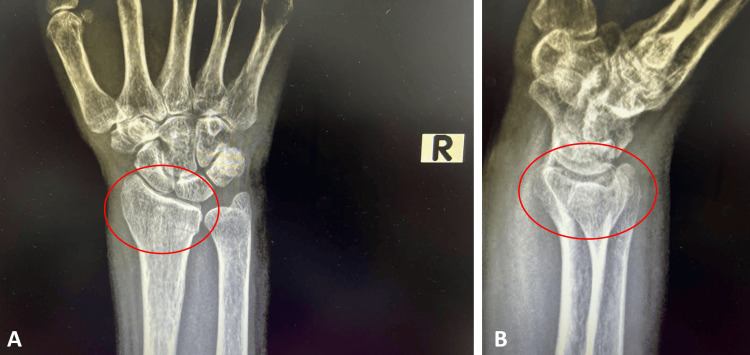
The wrist radiographs of the patient before splint application Wrist anteroposterior (1A) and lateral (1B) views of the patient prior to splint application revealed a nondisplaced fracture of the distal radius
